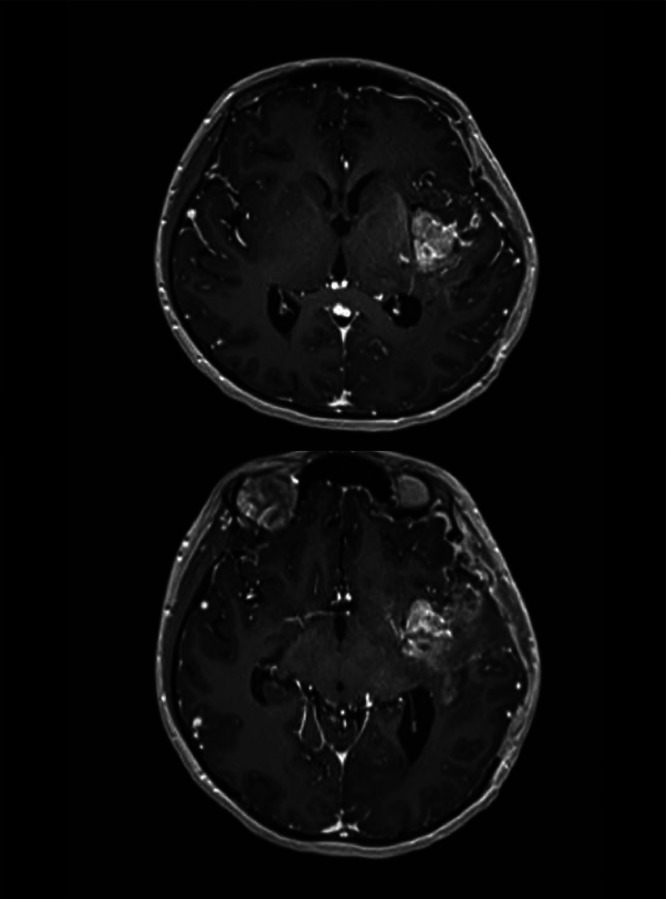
The first MRI image postoperative. The MRI shows a left frontotemporal lesion that appears hyperintense on T1 fat-saturated and T2-weighted images, with diffusion restriction suggestive of a solid lesion. After gadolinium contrast enhancement, heterogeneous enhancement of the lesion is observed, which suggestive of a probable aggressive pathology.
